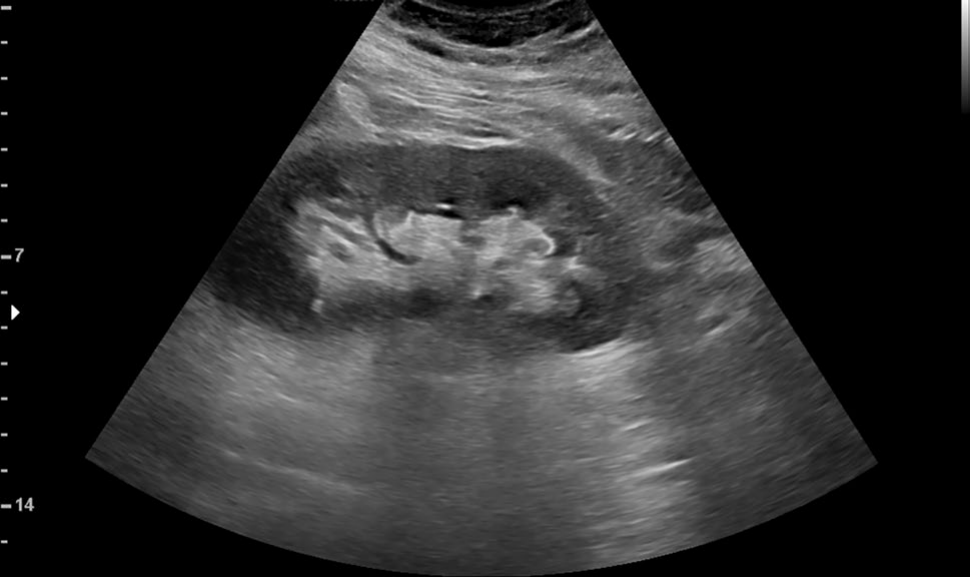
B-mode ultrasound. Left kidney with a normal cortical medullary representation of the parenchyma. No perirenal fluid or subcapsular hematoma were appreciable. Non parenchymal lesions or intraparenchymal fluid collections were detected
